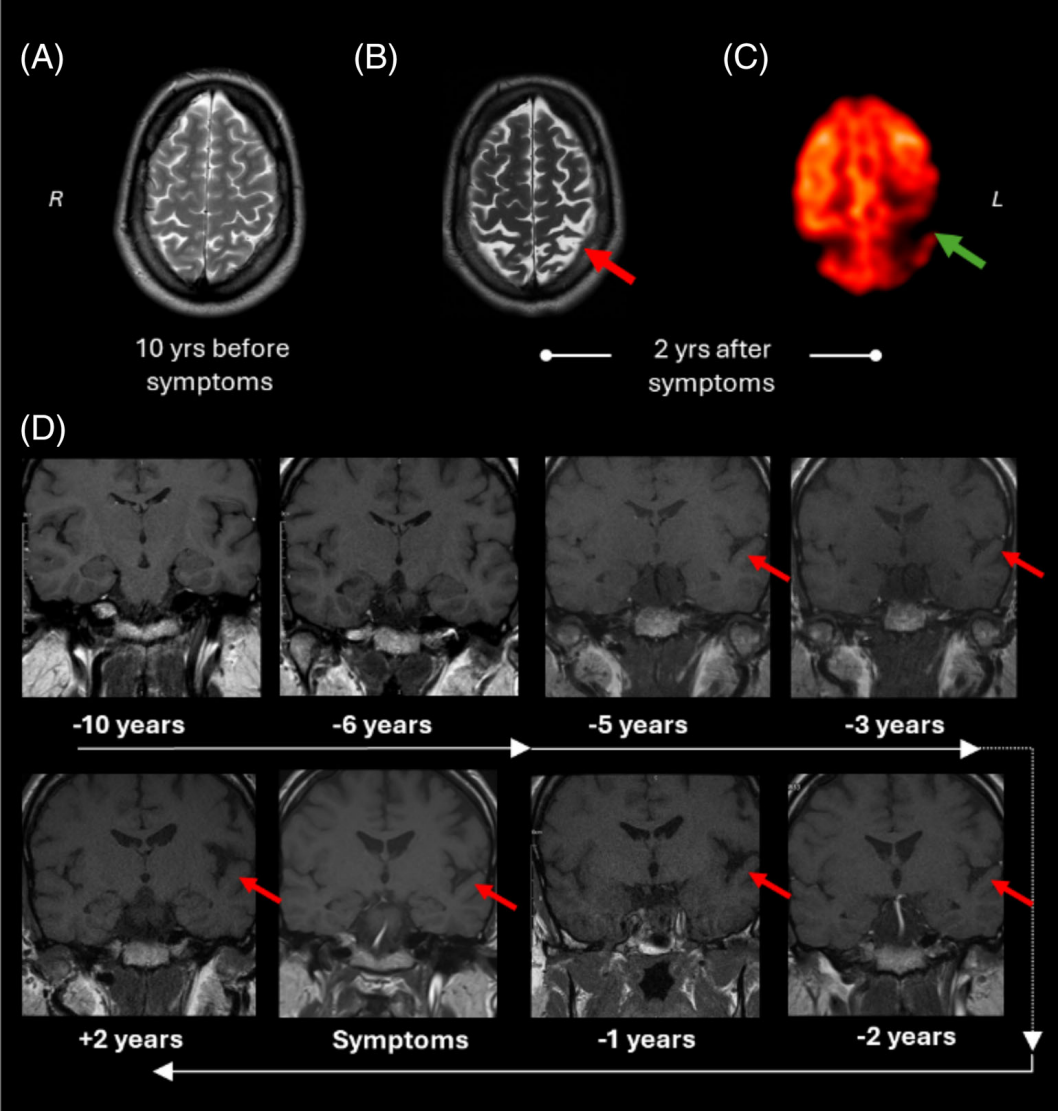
Brain MRI, axial T_2_‐weighted images, and axial 18F‐FDG PET images of the patient. (A) Mild generalized atrophy with a slight parietal predominance 10 years prior to symptom onset. (B) At the time of presentation to clinic, 2 years after symptom onset, there is significant atrophy with disproportionate, asymmetrical (left > right) parietal atrophy (red arrows). (C) Asymmetric hypometabolism in left parietal region at the time of presentation (green arrow). (D) A decade of annual coronal T_1_‐weighted images acquired to follow up the pituitary tumor show mild generalized atrophy 10 years prior to symptom onset with progressive volume loss. Asymmetric atrophy becomes evident up to 5 years before symptoms manifesting as widening of the left sylvian fissure on these images. MRI, magnetic resonance imaging; PET, positron emission tomography. [Color figure can be viewed at wileyonlinelibrary.com]
